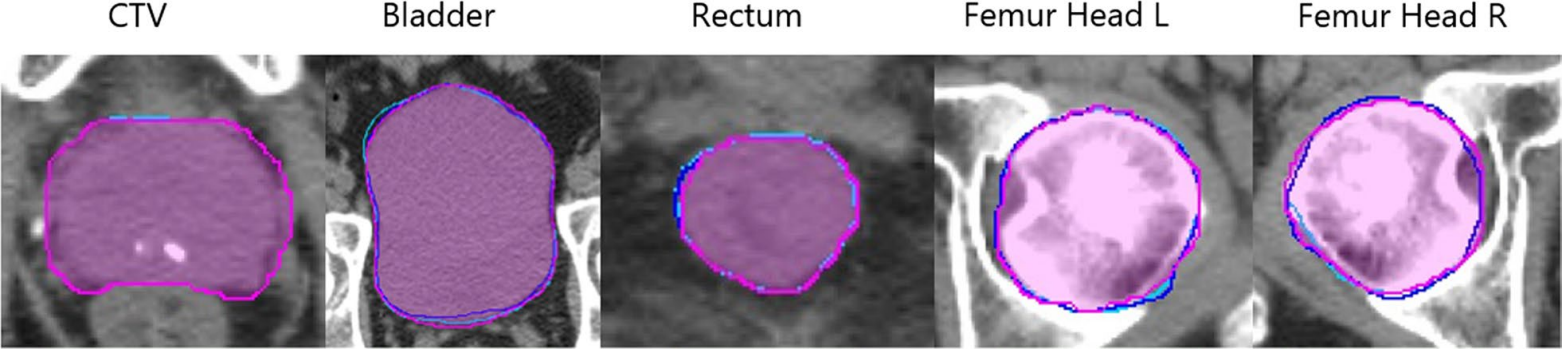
Examples of contours transferred to reference planning CT images. Purple colorwash: contours on planning CT image; Dark Blue line: contours on T2 HSLQ image; Sky blue line: contours on the T2 synLSHQ image
